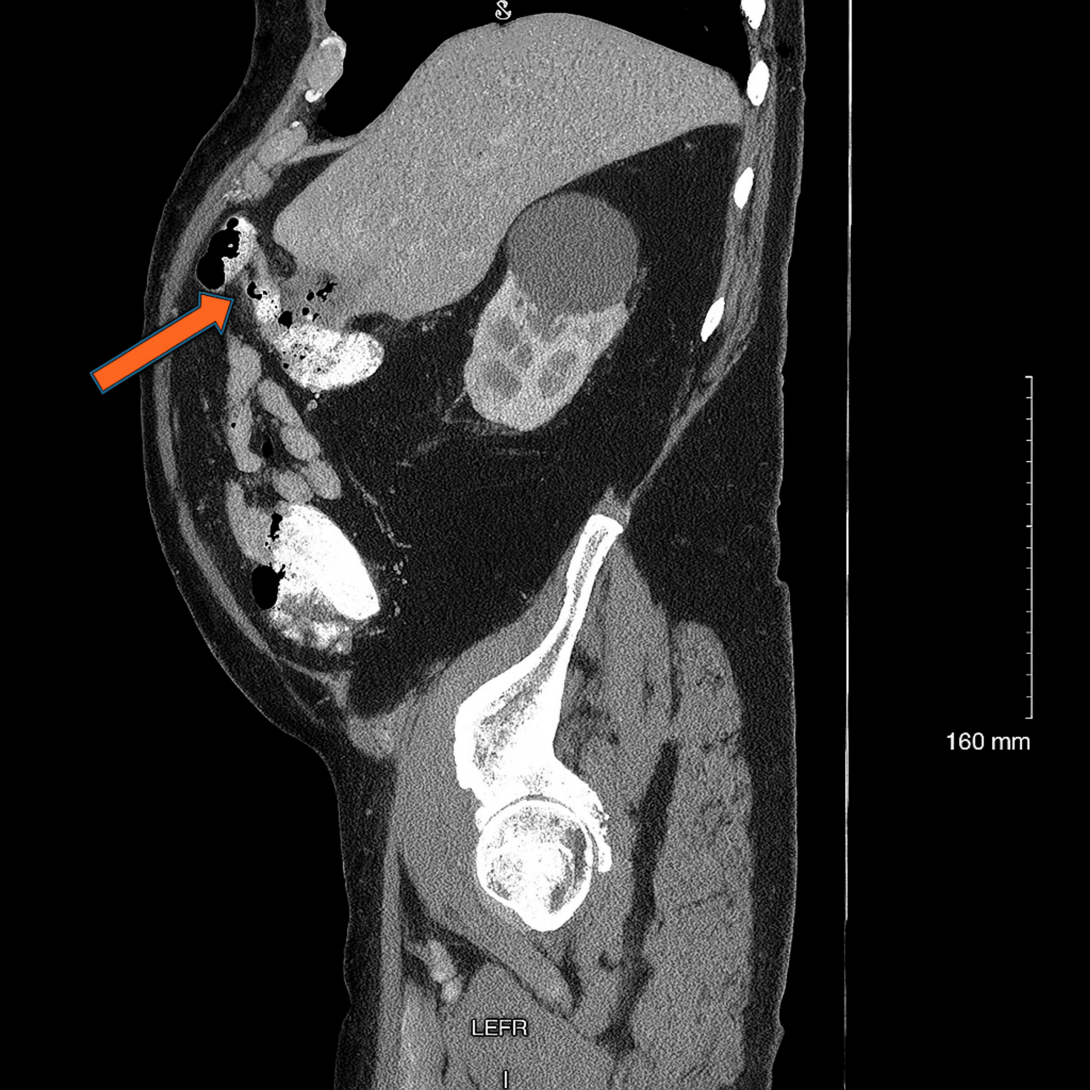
Sagittal computed tomography image with intravenous contrast providing an additional view of the fistula and pneumobilia.
